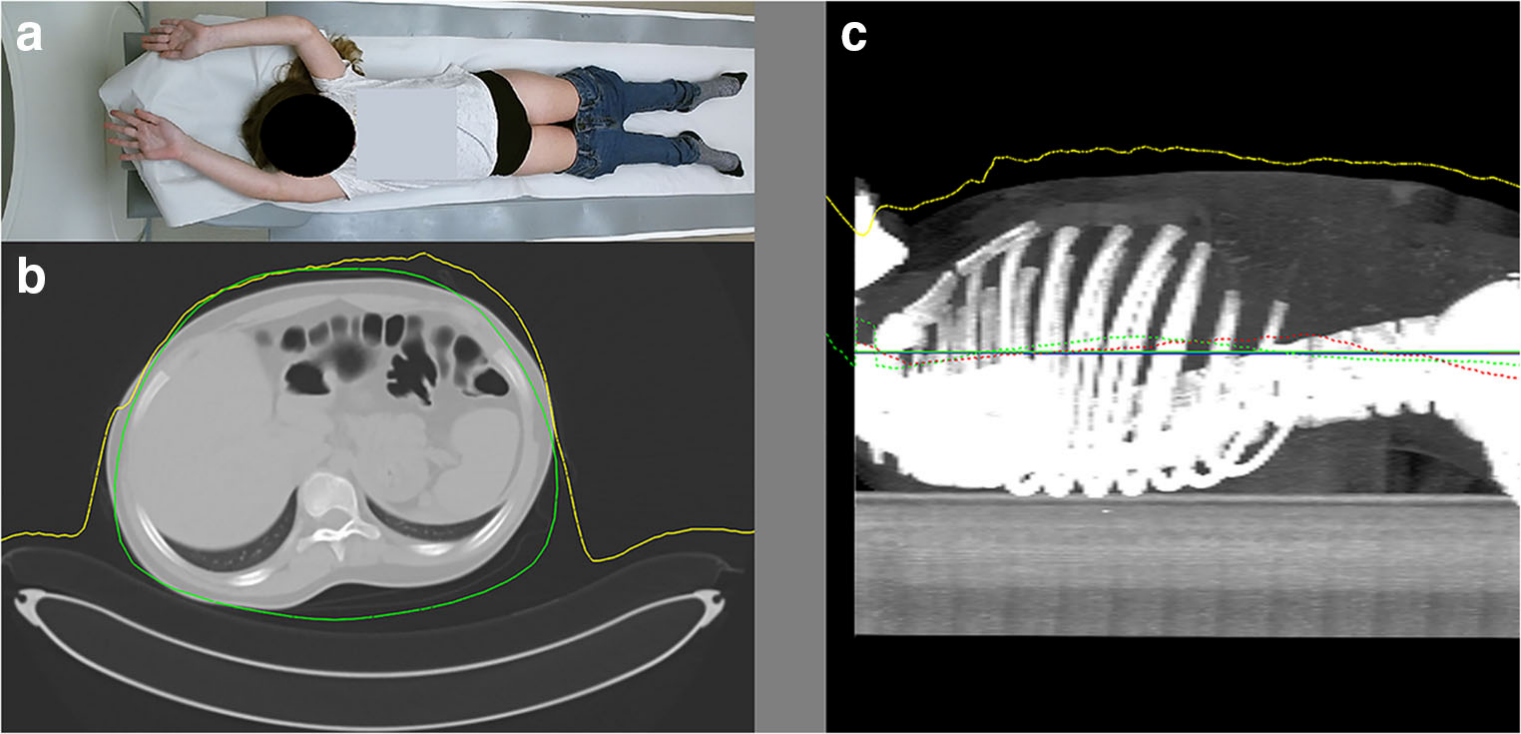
Case presentation of a 12-year-old child. a Color image taken by the 3D camera system. b Axial image of the abdomen with depth measurements (yellow line) by the 3D camera and the body contour (green) estimated by the algorithm. c Sagittal image of the thorax-upper abdomen with patient positioning accuracy: blue horizontal line: patient isocenter estimated by the radiographer, green horizontal line: average patient isocenter estimated by the camera, green dotted line: Avatar isocenter curve, red horizontal line: average patient isocenter (ideal table height), red dotted line: patient isocenter per axial cross-section, yellow line: depth measurements. The red, green, and blue straight lines are hard to distinguish from each other due to almost similar values as the ideal table height
